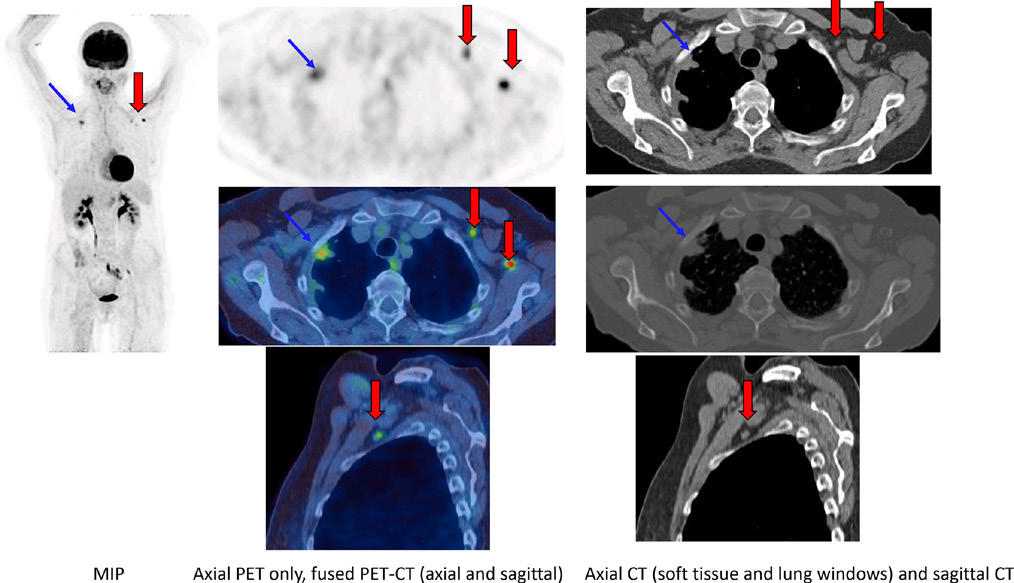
FDG PET-CT (MIP, axial PET only, fused PET-CT, CT only in lung and soft tissue windows, sagittal fused PET-CT and CT only) demonstrated intensely avid uptake in the right apex in keeping with a primary lung malignancy. Intense uptake was seen in normal-sized left axillary and pectoral lymph nodes with a normal fatty hilum.
